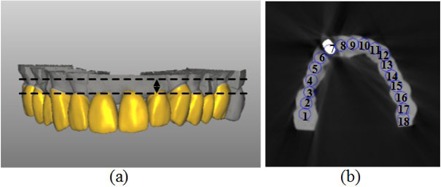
Four slices of a maxillary cast (a) were selected. A slice of the image sets of the 7th experiment (b) and the setting of the 18 ROIs for each slice in CBCT image sets.
